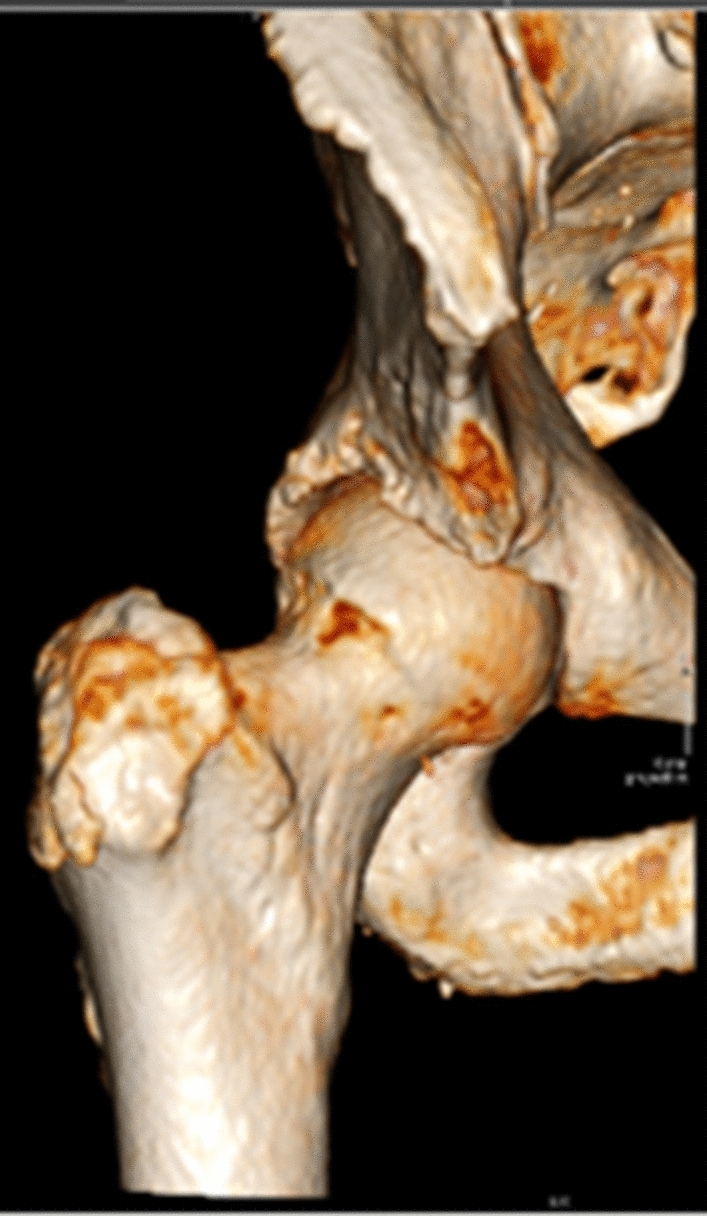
3D computed tomography (CT)
